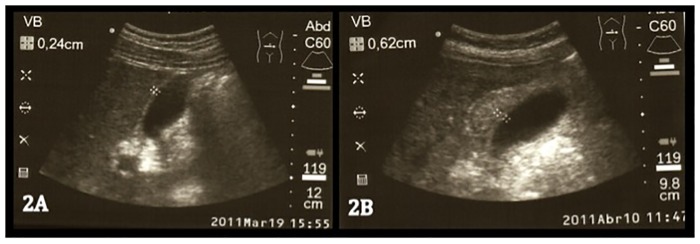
Abdominal ultrasound in a control patient (2A) and in a patient with severe dengue (2B).
